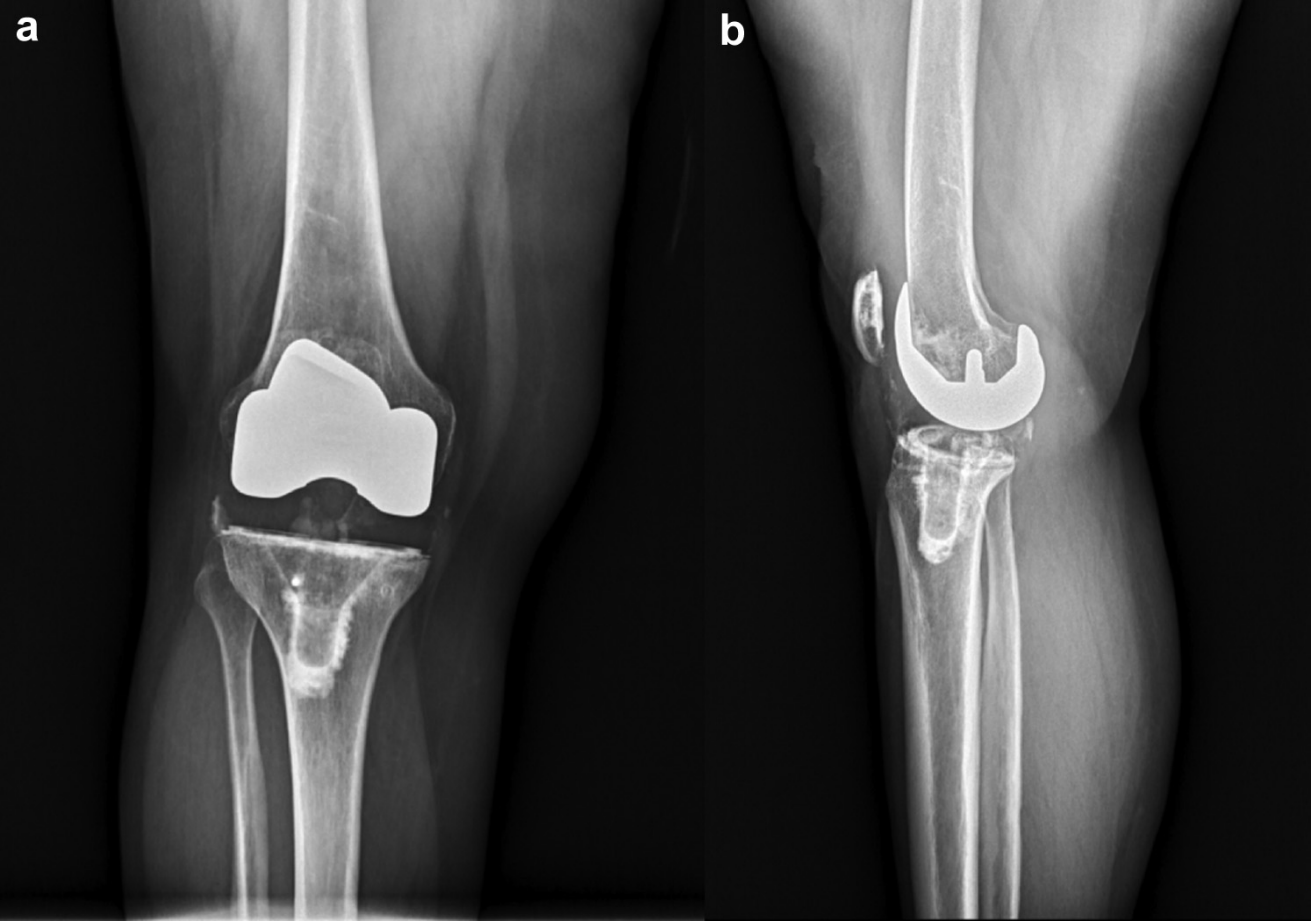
(a) Anteroposterior and (b) lateral radiographs of the right knee of case 1 demonstrate cemented right total knee arthroplasty with Stryker Triathlon cruciate retaining femur and all-polyethylene tibia in good alignment without fracture, subsidence, loosening, or osteolysis.
